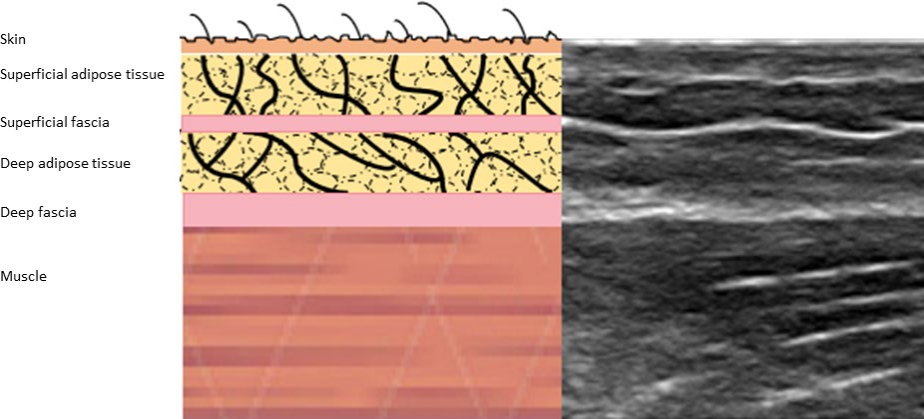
Depiction of the structural layers from the skin to the skeletal muscle as seen in the ultrasound image.
